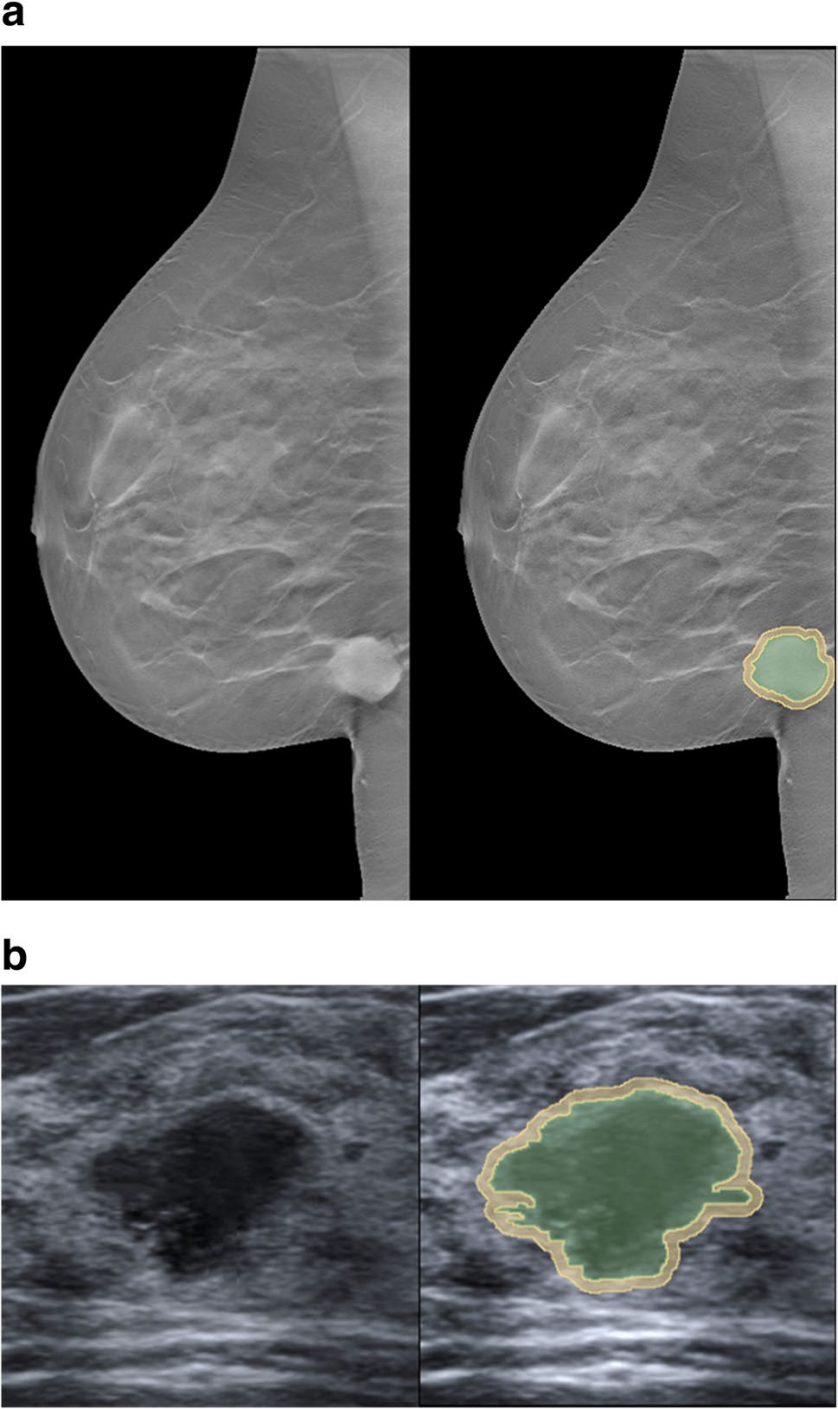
Examples of segmentation in tomosynthesis and ultrasound images. a Tomosynthesis. b Ultrasound. Tumor segmentations (green) were delineated semi-automatically; peritumor segmentations (yellow) were generated by “Hollow” effect
